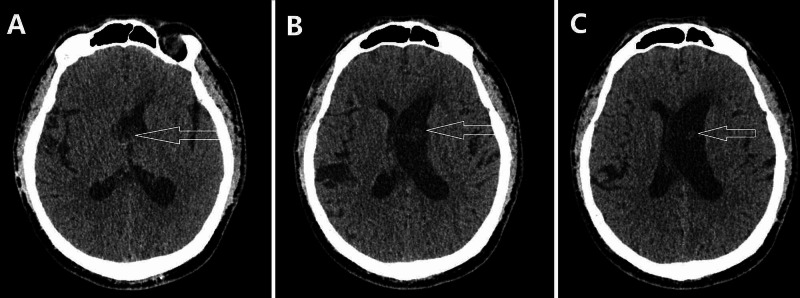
Axial computed tomography. Axial computed tomography scan revealing an intraventricular low-density lesion without a calcification mass attached to the anterior third of the septum pellucidum and Monro's foramen and enlargement of the left lateral ventricles.
